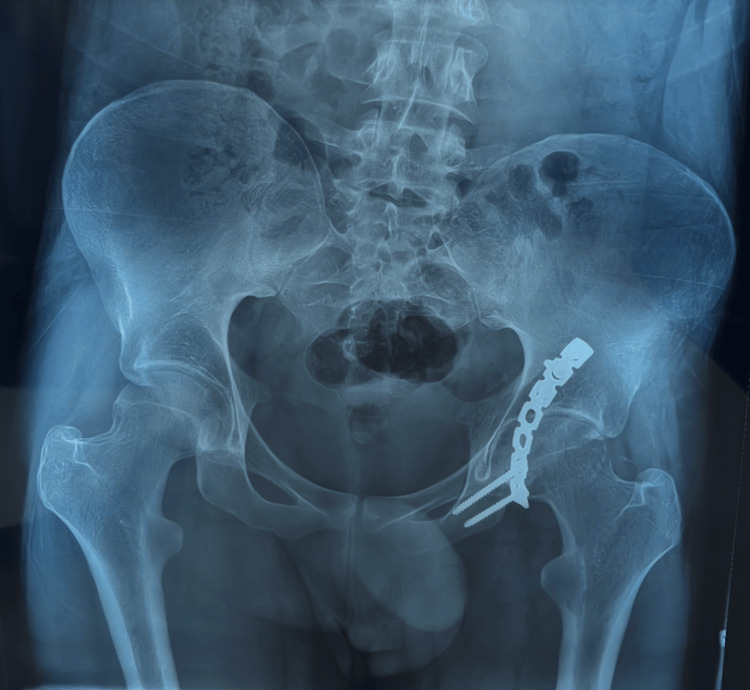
Postoperative pelvic X-ray showing plate and screw fixation of a posterior wall acetabular fracture. The posterior approach places the sciatic nerve in close proximity to the surgical field, highlighting the risk of iatrogenic sciatic nerve injury in this anatomical context.
